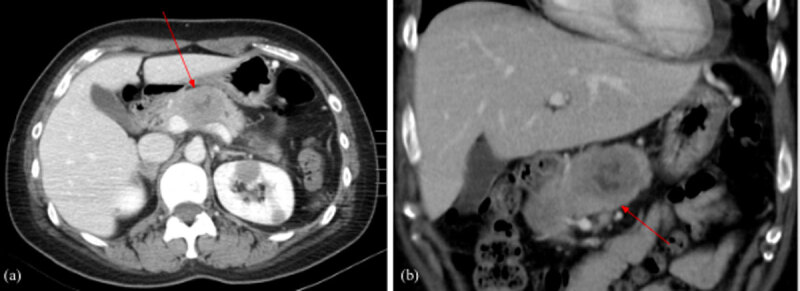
Contrast-enhanced abdominal CT scan axial (a) and coronal (b) views: Poor enhancing mass lesion at the body of pancreas with encasement of the superior mesenteric vein.
